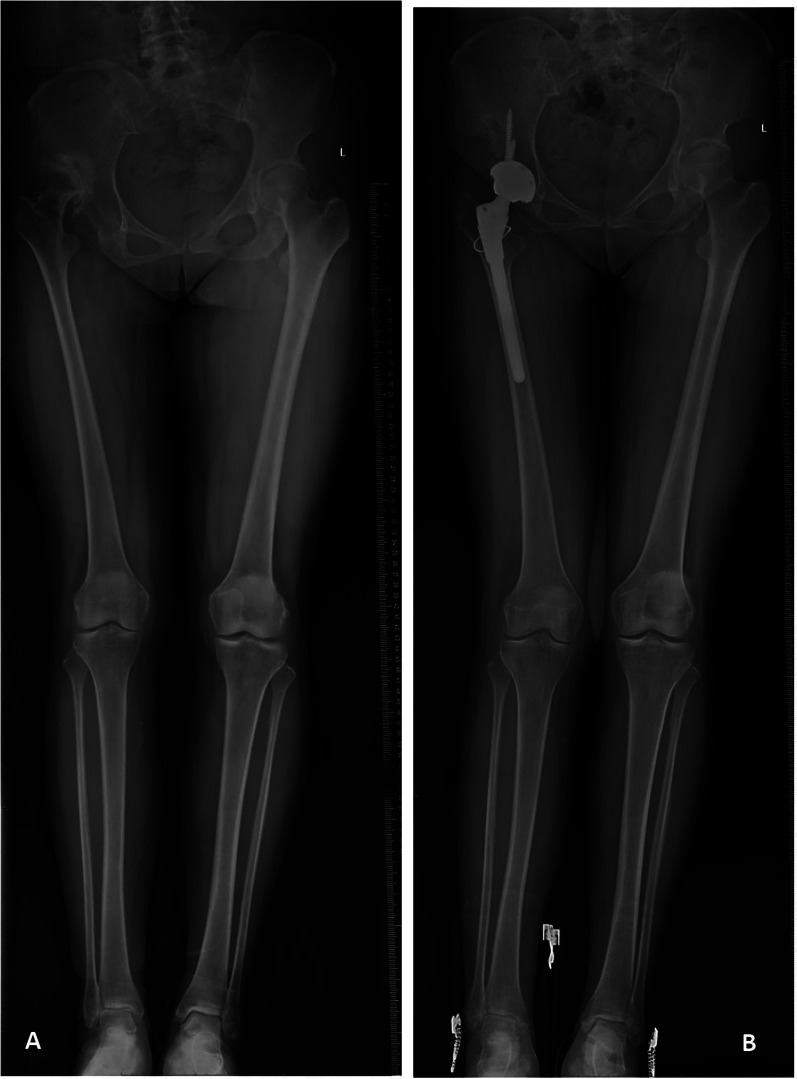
Valgus alignment of the knee in a 54-year-old Crowe type IV patient after total hip arthroplasty. A Preoperative radiograph. B Postoperative radiograph
